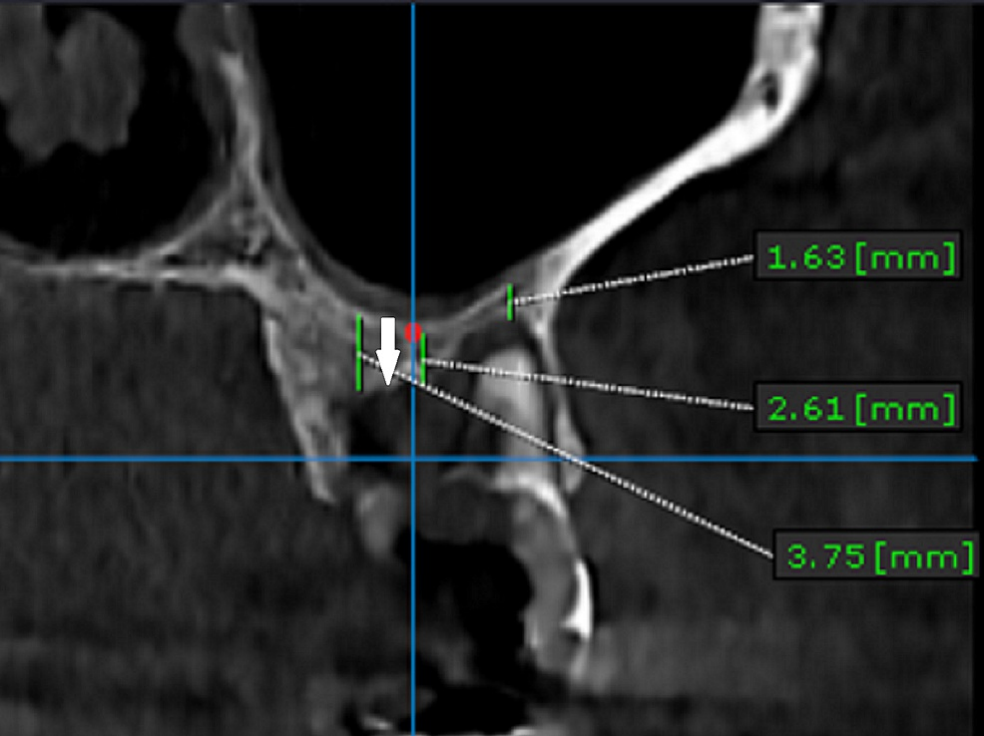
CBCT scan of the left upper first molar region taken preoperatively before the surgical procedure. The arrow denotes the distance from the sinus lining to the alveolar basal bone, and the distances are different at different points of the tooth involved. CBCT: cone-beam computed tomography
